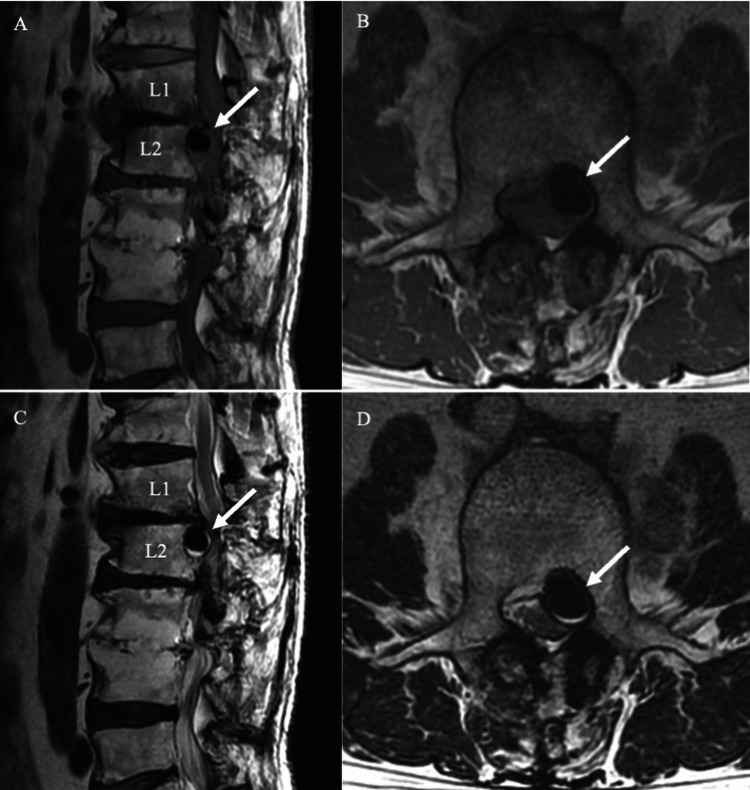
Progressive Enlargement of Gas-Containing Herniation Sagittal and axial T1-weighted (A, B) and T2-weighted (C, D) MRI scans obtained 18 days before surgery show significant enlargement of the epidural gas-containing lesion at L1/2 *(white arrow)*. The lesion compresses the thecal sac and the left L2 nerve root.
